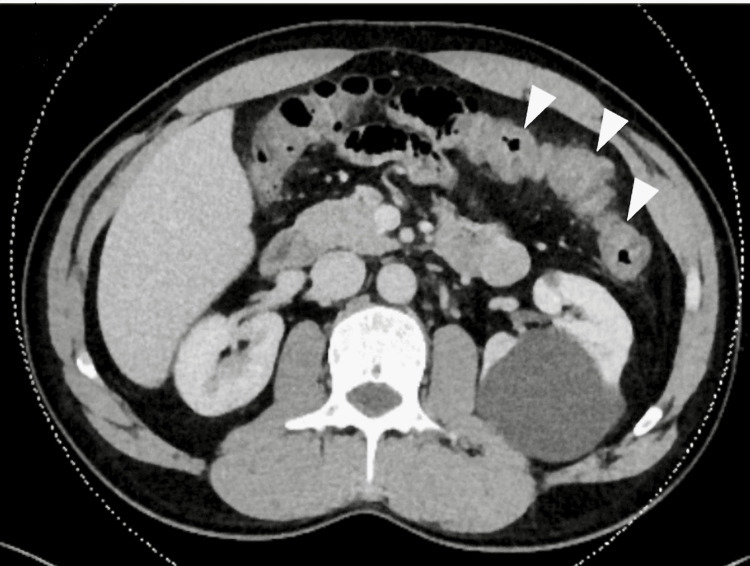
Computed tomography (CT) scan of the abdomen showing colonic inflammation Colitis is indicated by spearhead arrows.
